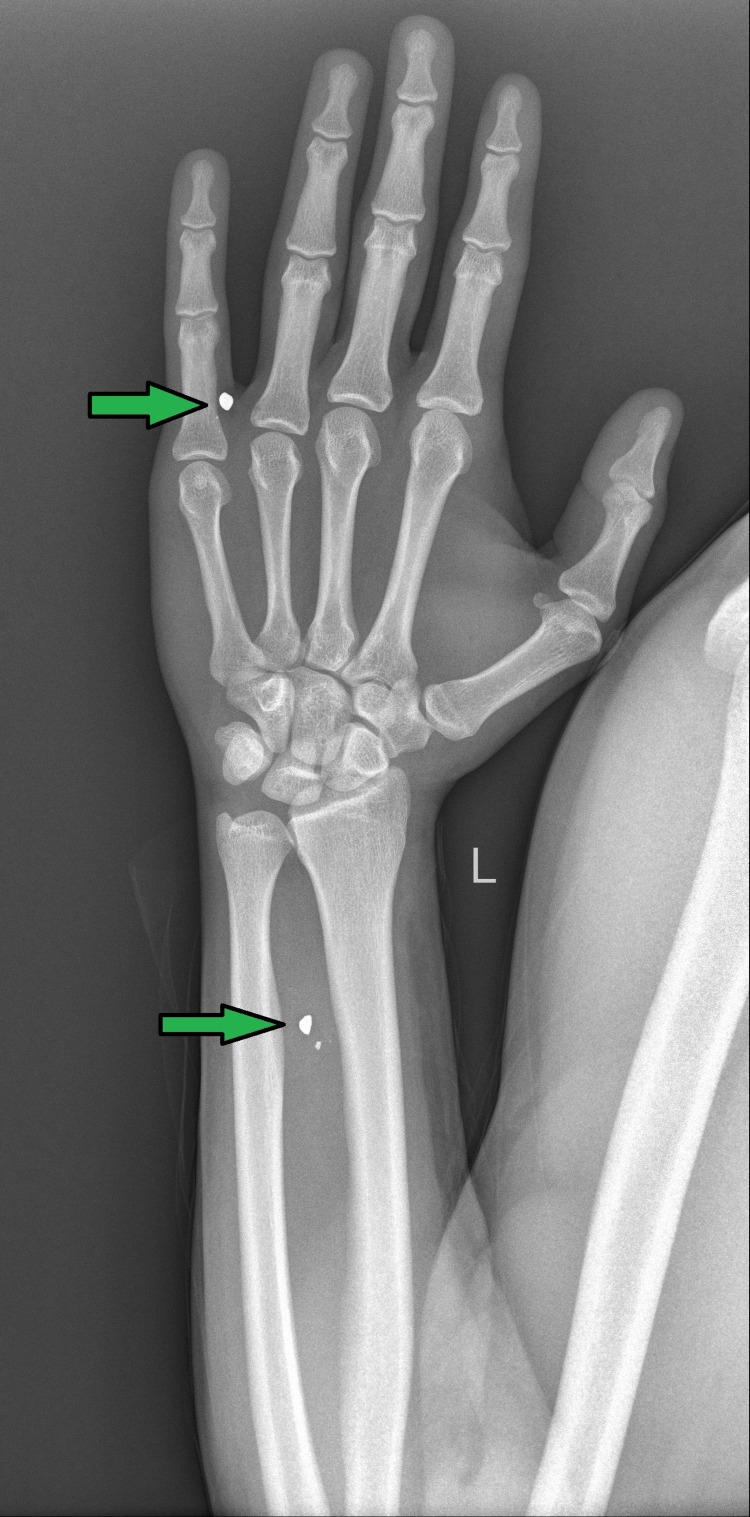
X-ray AP view of the left wrist showing tiny pellets seen in the lower one-third of the left forearm and in between the fourth and fifth proximal phalanges. In comparison with the previous X-ray, there are no significant interval changes AP: anteroposterior
